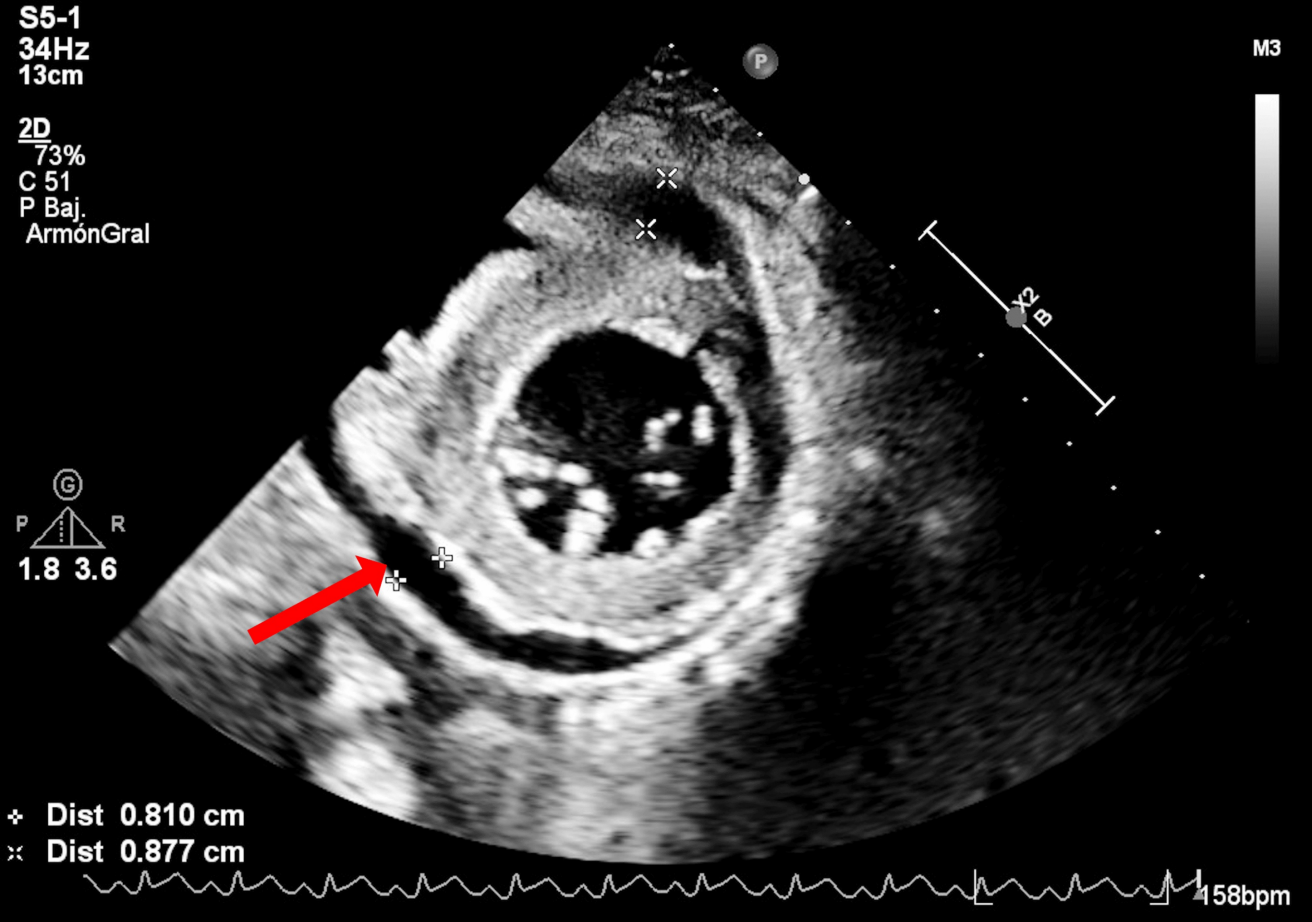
Echocardiogram: parasternal short axis view Pericardial effusion and hyperechogenic myocardium due to inflammation and edema were noted.
